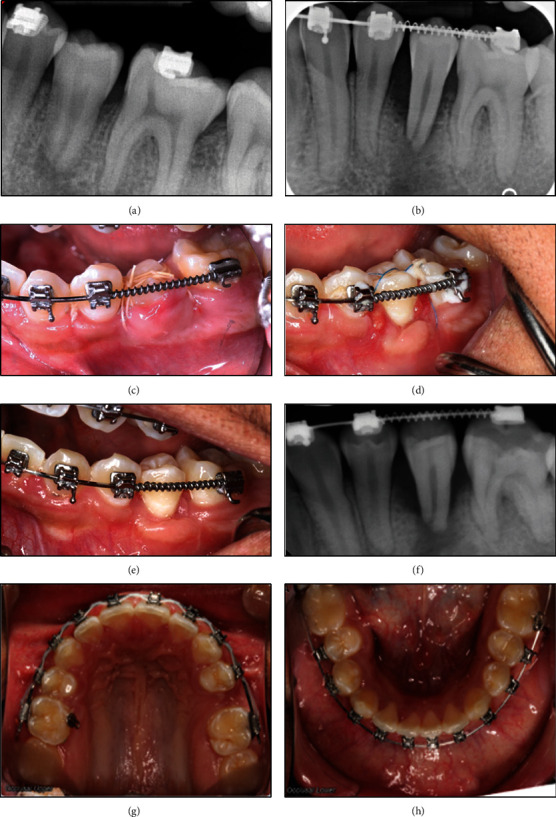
Post-operative records of auto-transplantation. (a) Post-operative periapical radiograph of immediate auto-transplantation of tooth #13 (donor tooth) to the socket of tooth #20 (recipient site). (b) Periapical radiograph 1-week follow-up of auto-transplantation of tooth #20. (c) Clinical photo 1-week post-operative of auto-transplantation of tooth #20. (d) Clinical photo 3-week post-operative of auto-transplantation of tooth #20. (e) Clinical photo 6-week post-operative of auto-transplantation of tooth #20. (f) Periapical radiograph showing 6-week post-operative follow-up of auto-transplantation of tooth #20. (g) Clinical photo 6-week post-operative of auto-transplantation: maxillary occlusal view. Teeth #4 and #13 were donor teeth, and spaces were closing. (h) Clinical photo 6-week post-operative of auto-transplantation: mandibular occlusal view. Teeth #20 and #29 were recipient sites, and spaces were closed.
